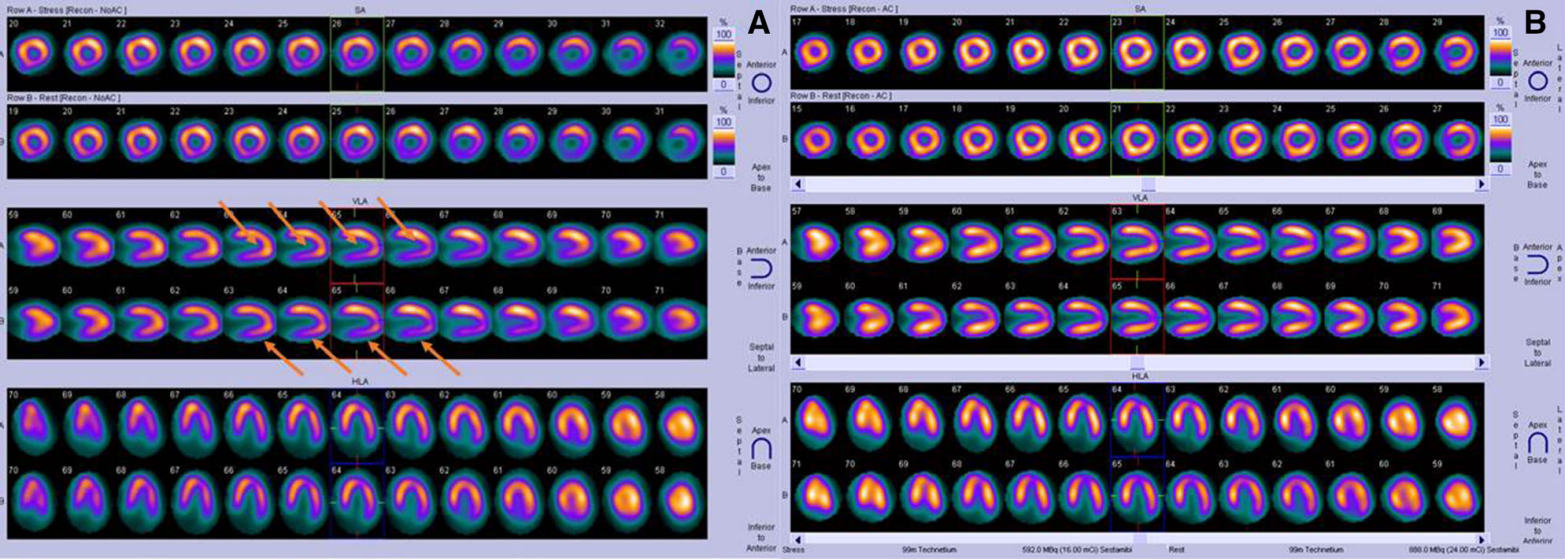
In MIBI SPECT/CT no reversible perfusion defects were found. Moderate fixed perfusion defects in inferior wall of left ventricle were seen in images without attenuation correction (red arrows). After correction of attenuation—the inferior wall perfusion was normalized. (A) Myocardial perfusion MIBI SPECT/CT—study without attenuation correction. (B) Myocardial perfusion MIBI SPECT/CT—study with attenuation correction
